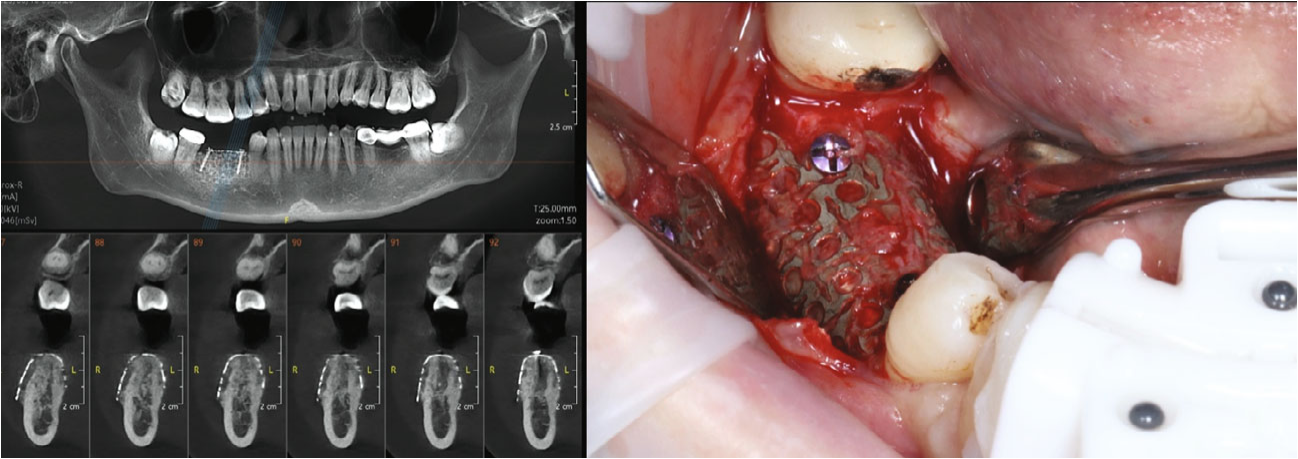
Radiographic and clinical images during the re-entry for implant placement in Positions 4.5 and 4.6. Notice the substantial bone regeneration achieved.
